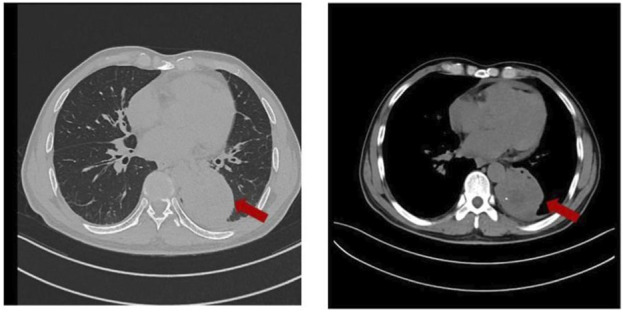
Chest CT scans showed the primary lesions (red arrowheads) in the lung on 24 December 2024.
